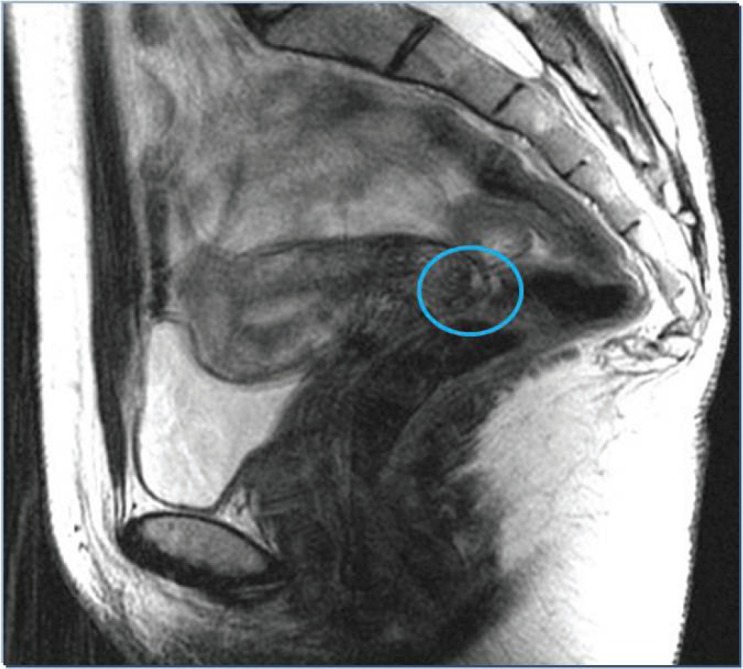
MRI: 25-mm cervical lesion with no infiltration into the parametria.
